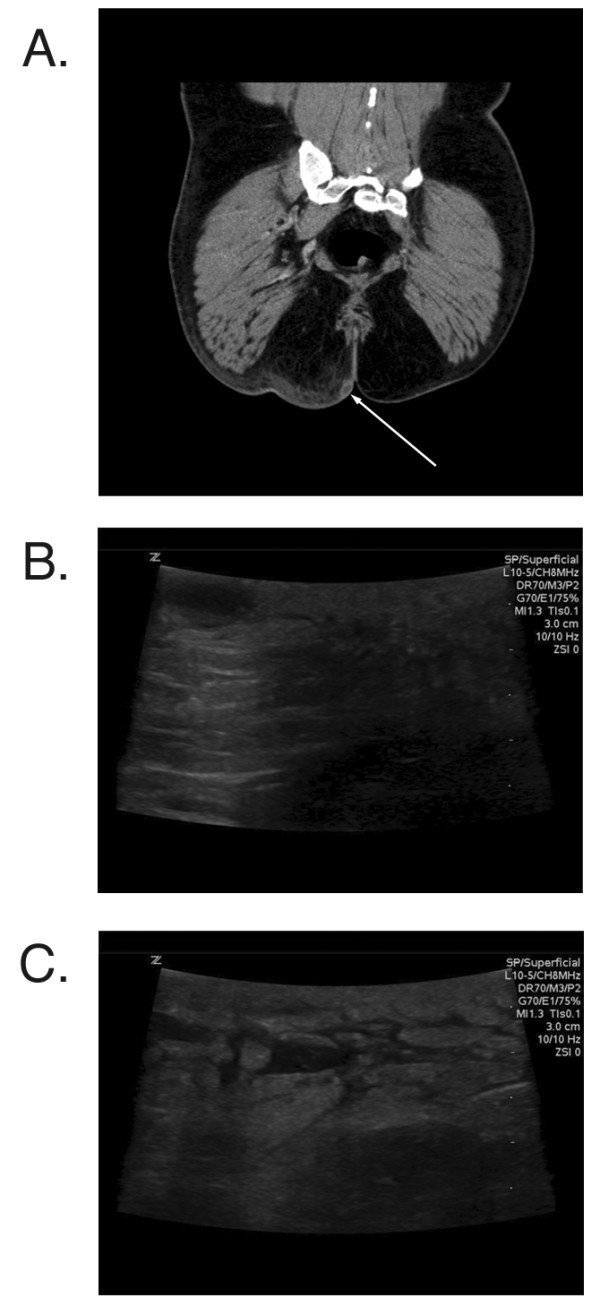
US and CT of patient with buttock abscess. Figure (A) demonstrates CT imaging of a patient with a buttock abscess. Note the stranding in the soft tissue with small central abscess cavity (white arrow). (B) demonstrates sonographic imaging of the same patient (long axis orientation) with visualized debris in the dependent portion of the abscess cavity and posterior enhancement deep to the abscess cavity. (C) demonstrates sonographic imaging of tissue lateral to the abscess cavity with branching anechoic wisps surrounding small islands of hyperechoic tissue.
